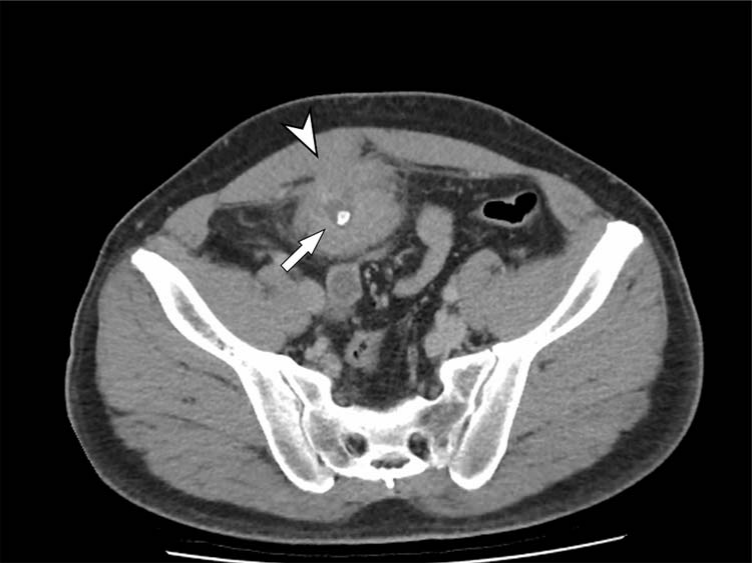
Axial CT image of the pelvic lesion. Arrow indicates central calcification. Arrowhead indicates involvement of anterior abdominal wall.
